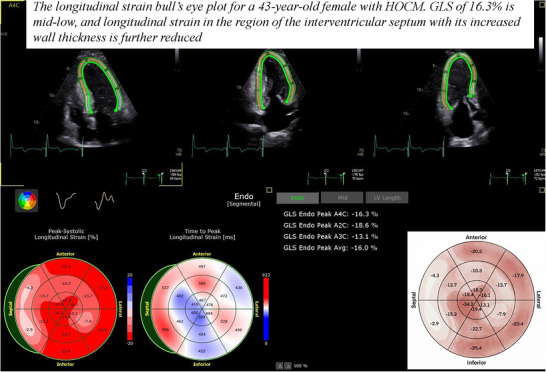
Representative LV longitudinal strain in HOCM. Apical 4‐, 2‐, and 3‐chamber speckle‐tracking views with corresponding bullseye plot demonstrate heterogeneous peak systolic longitudinal strain, with relatively reduced septal strain compared with other segments, despite only mildly reduced global longitudinal strain.
